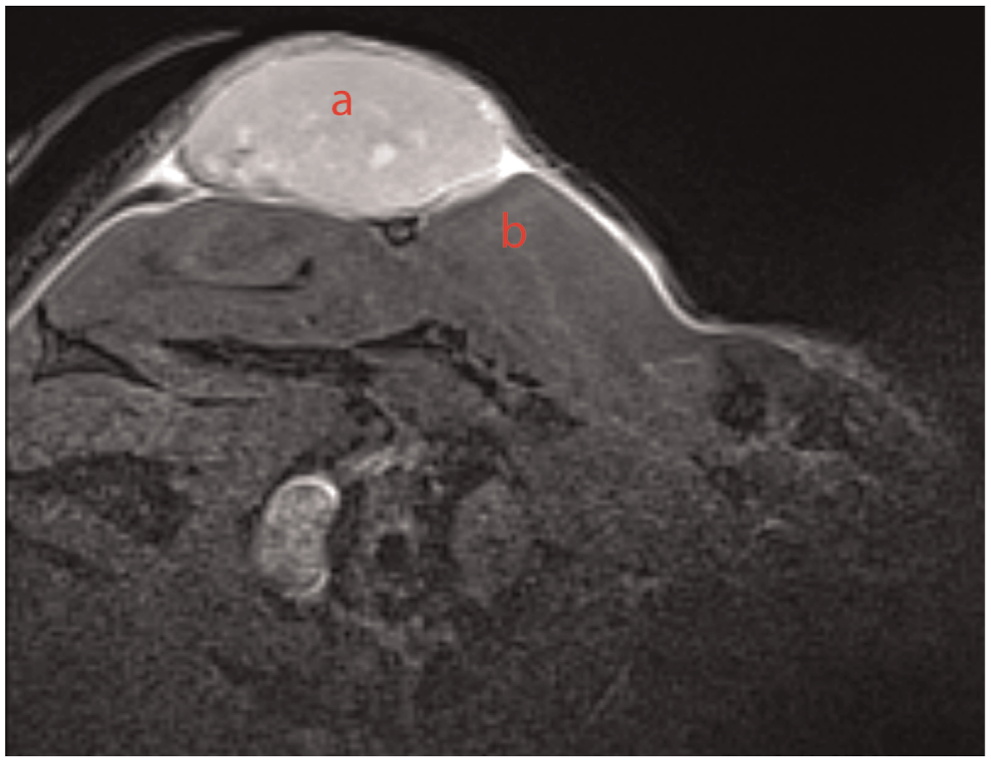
MRI of HT29 xenograft. HT29 colon carcinoma (a) grown at the subcutaneous injection site above muscles (b) appear hyper-intense in two-dimensional turbo spin-echo (TSE) sequence (MR images in axial orientation).
